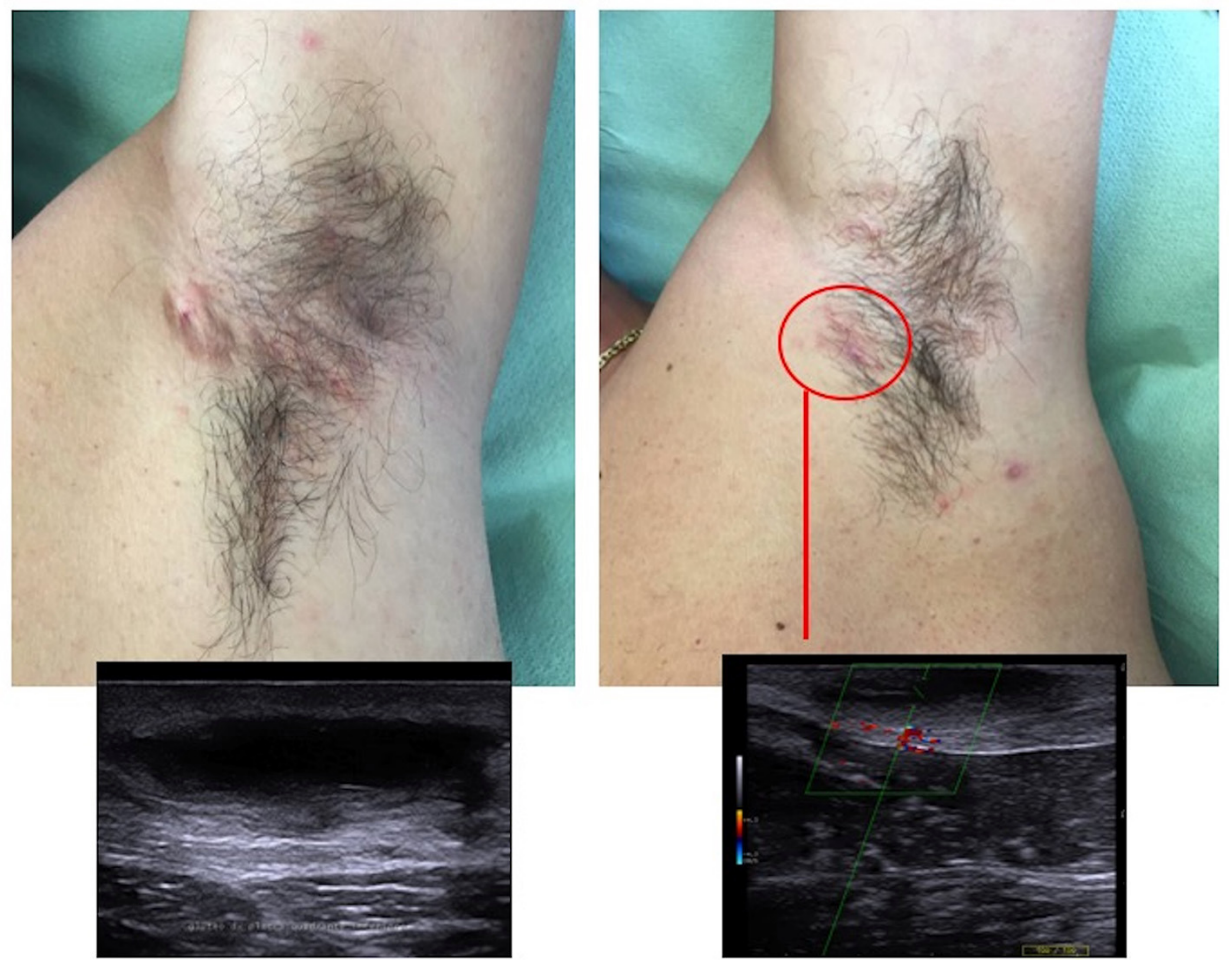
Hidradenitis suppurativa lesions of the armpit before and after cryotherapy, with ultrasound assessment documenting improvement, but the persistence of a pseudocyst hypoechoic nodule with the fibrotic surrounding tissue.
